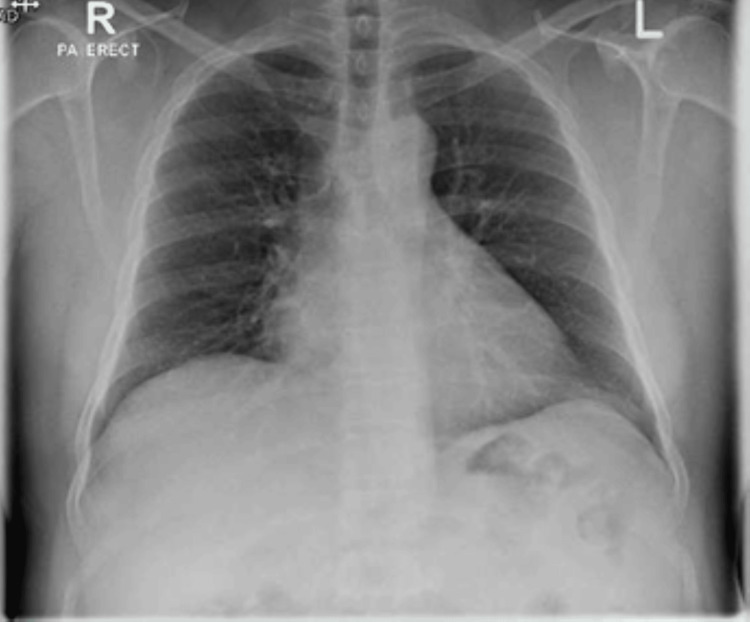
Normal chest X-ray. PA: posterior to anterior.
